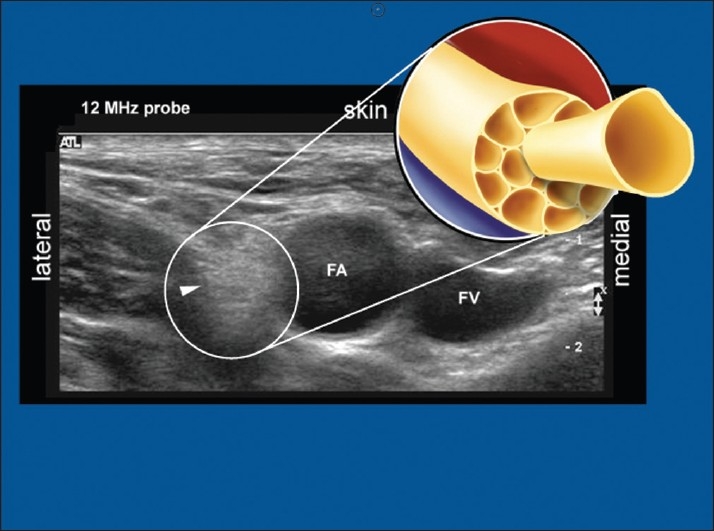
Ultrasound appearance of the femoral nerve – a peripheral nerve. Note the nerve appears hyper-echoic
